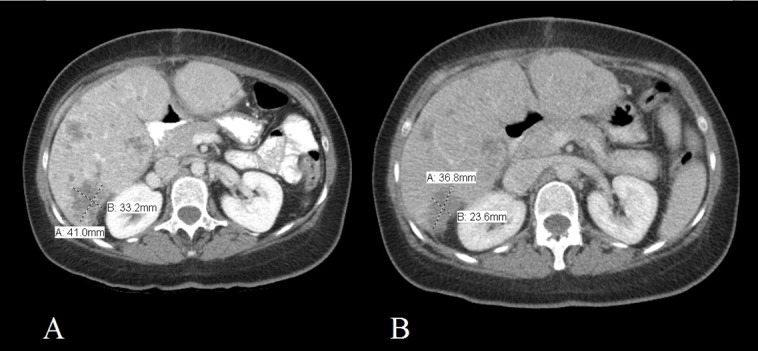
Figure showing the computed tomography of the abdomen with IV contrast Images taken one month before (A) and five months into treatment (B) with estradiol
